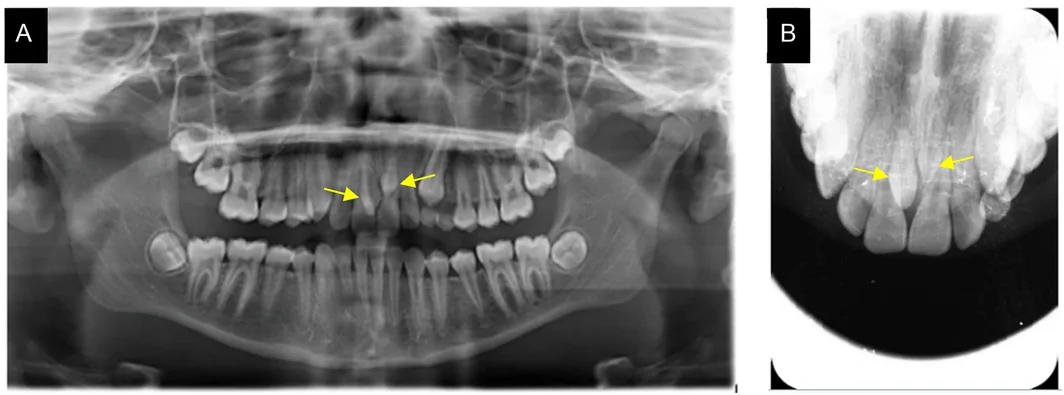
(A) Orthopantomograph showing full permanent dentition with retained maxillary left deciduous teeth (ULC and ULD) and supernumerary teeth present. (B) Upper standard occlusal radiograph showing the maxillary anterior dentition and supernumerary teeth.
